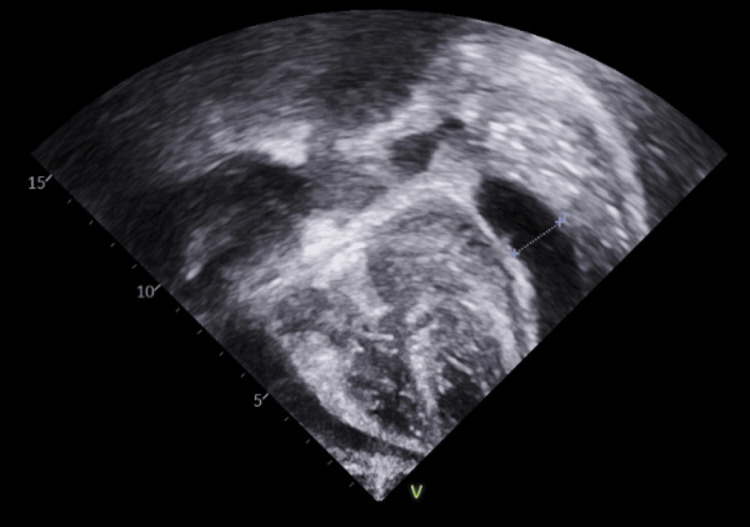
TTE showing pericardial effusion denoted by Xs and line TTE: transthoracic echocardiogram
